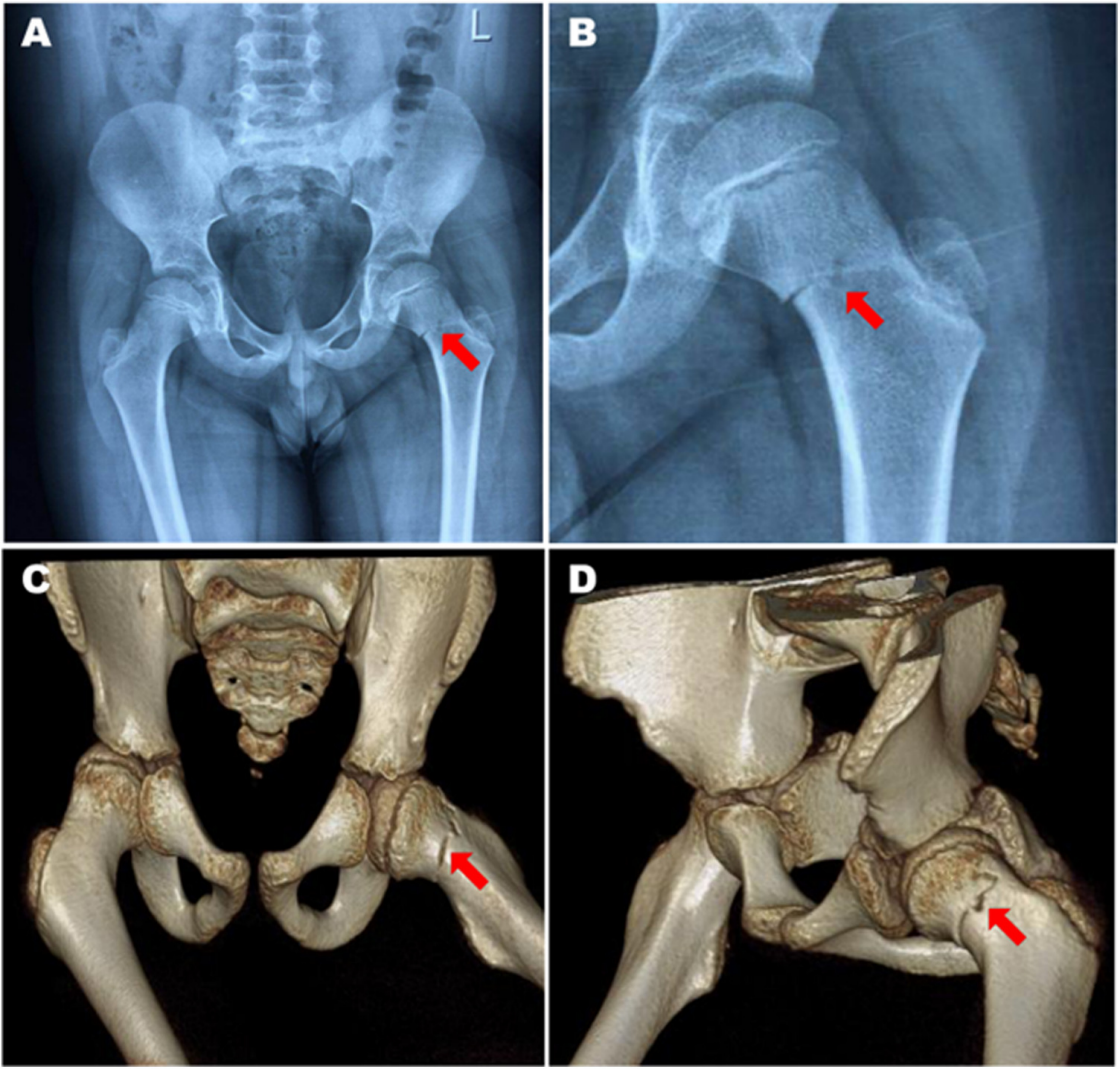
An 8-year-old male patient with Delbet-Colonna type II femoral neck fracture. (A,B) Preoperative x-rays showed minor displacement of the fracture with an indistinct fracture line. (C,D) The femoral neck fracture was confirmed by 3-dimensional computed tomography.
